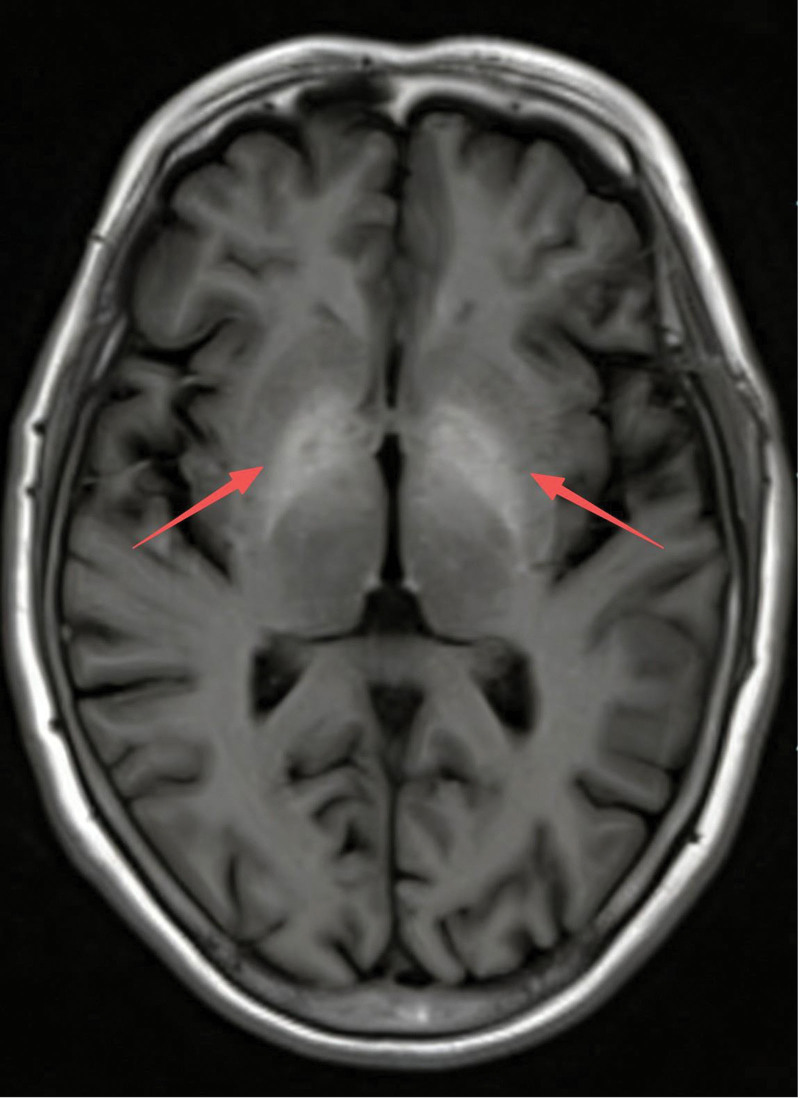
The brain MRI demonstrated bilateral T1 hyperintensity of the globus pallidus and posterior limb of the internal capsule. MRI = magnetic resonance imaging.
